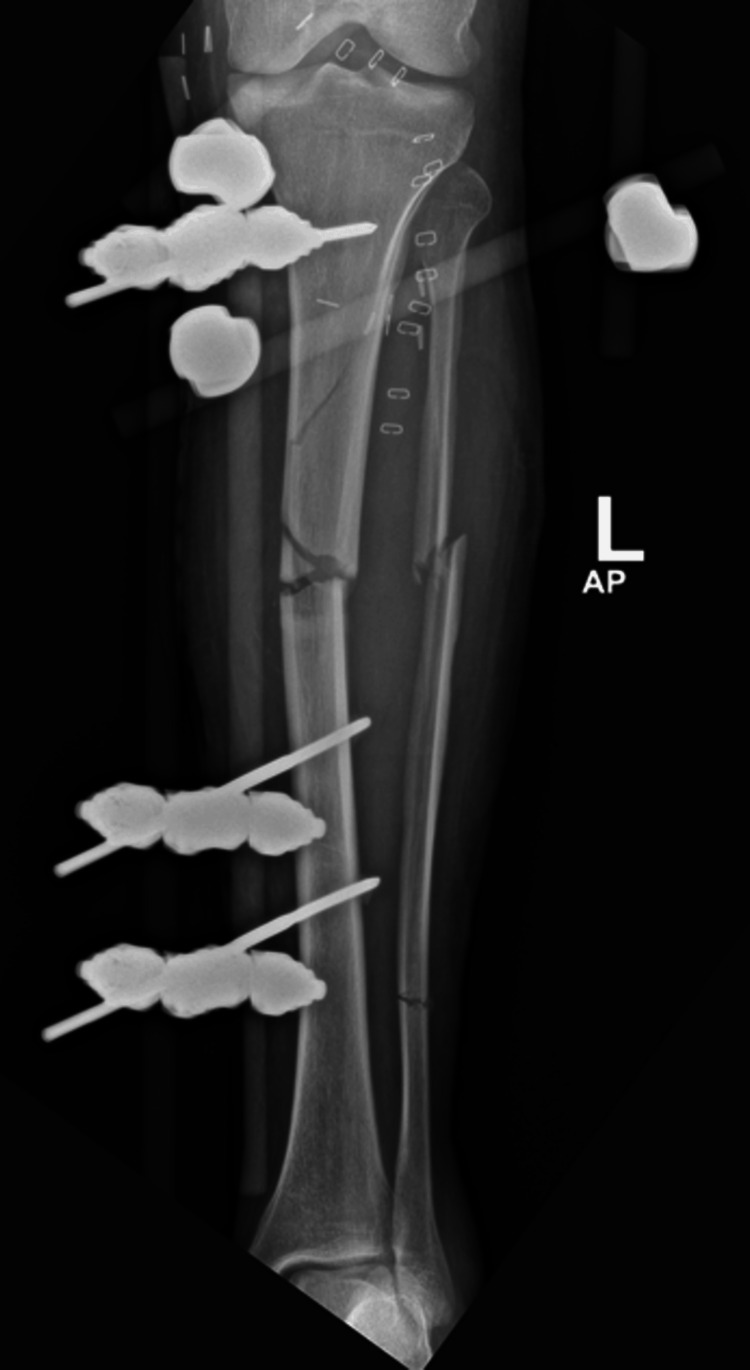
Postoperative anteroposterior radiograph of the left tibia and fibula showing an external fixator in place. The tibia demonstrated a comminuted fracture with a medial butterfly fragment and a fracture line extending to the proximal third. The fibula exhibits a segmental fracture.
